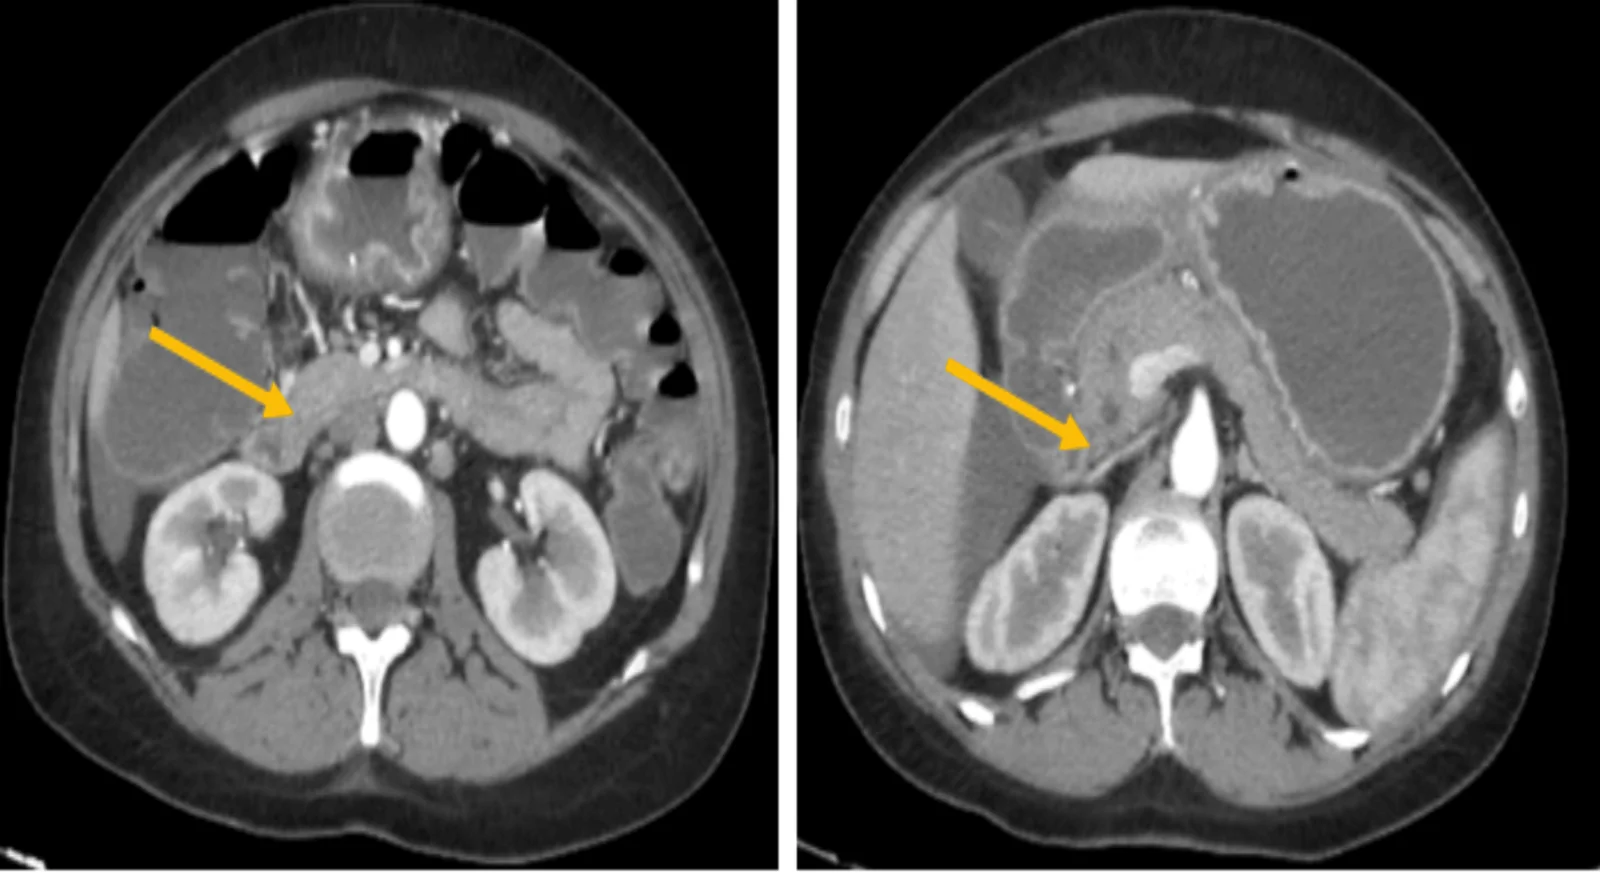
Abdominal CT showing acute pancreatitis (CTSI score, 2) Yellow arrow: pancreatic edema with peripancreatic fat stranding CTSI: CT Severity Index
